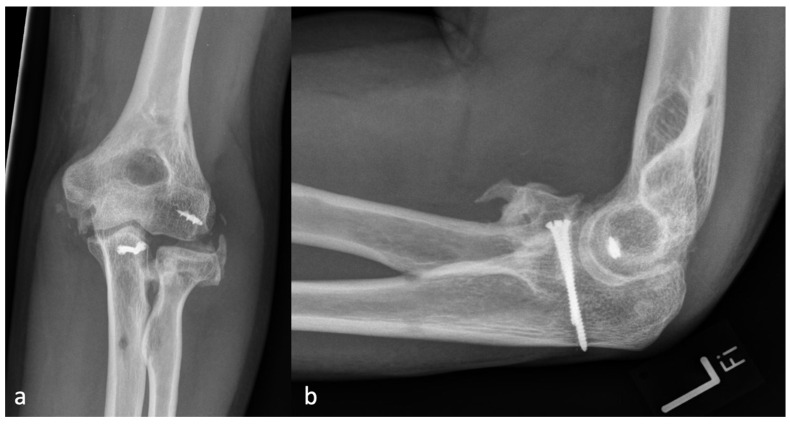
(a) shows the AP and (b) shows the lateral X-ray with a brace formation radio-ulnar and represents an HO 4.
